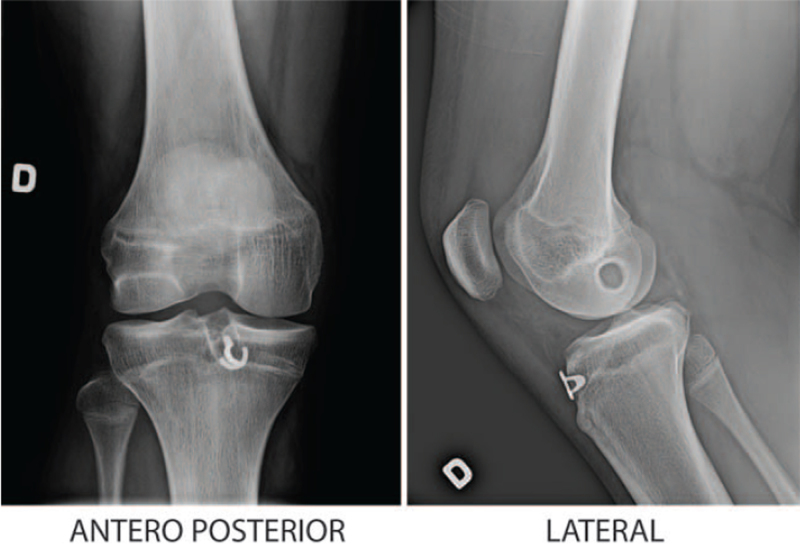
Radiographs of the largest tunnel enlargements measured after ACLR. The widest femoral tunnel diameter measurement was taken at the sclerotic margins of the bone. The same was done at the tibial tunnel. The Armorlink (Orthopediatrics Corp., Warsaw, IN) implant is visible at the tibia. Growth arrest lines are straight and well-defined, indicating no growth disturbance. Lateral left knee radiograph 13year-old boy with initial and final femoral tunnel sizes of, respectively, 7.5 mm and 11.2 mm. Anteroposterior left knee radiograph 13-year-old boy with initial and final tibial tunnel sizes of, respectively, 7.5 mm and 12 mm.
